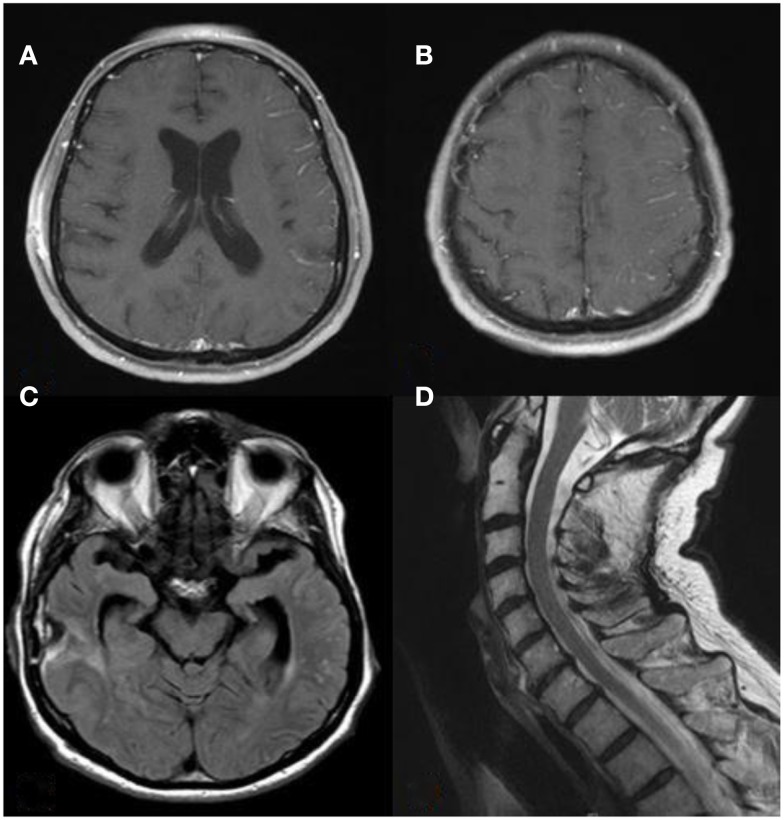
(A,B) T1 contrast-enhanced axial MRI shows new left frontal leptomeningeal enhancement. (C) T2 FLAIR shows new left temporal lobe changes. (D) T2 sagittal view of the cervical spine showed no evidence of intrinsic cord abnormalities.
